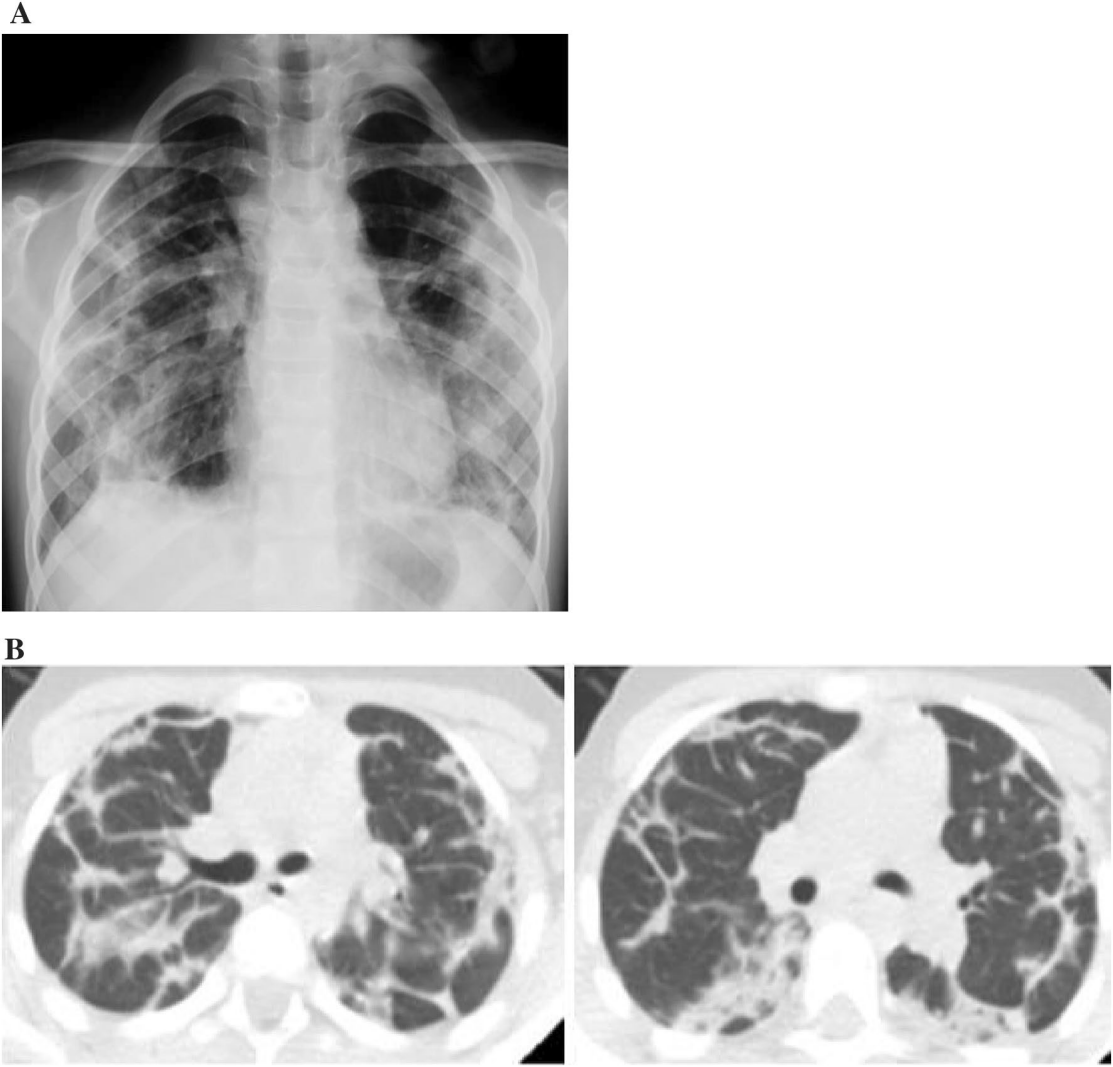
a Diffuse fibrotic changes and parenchymal consolidation on chest X-ray. b Common fibrotic changes, interlobular septal thickening, and subpleural parenchymal consolidation compatible with organising pneumonia on thorax CT
